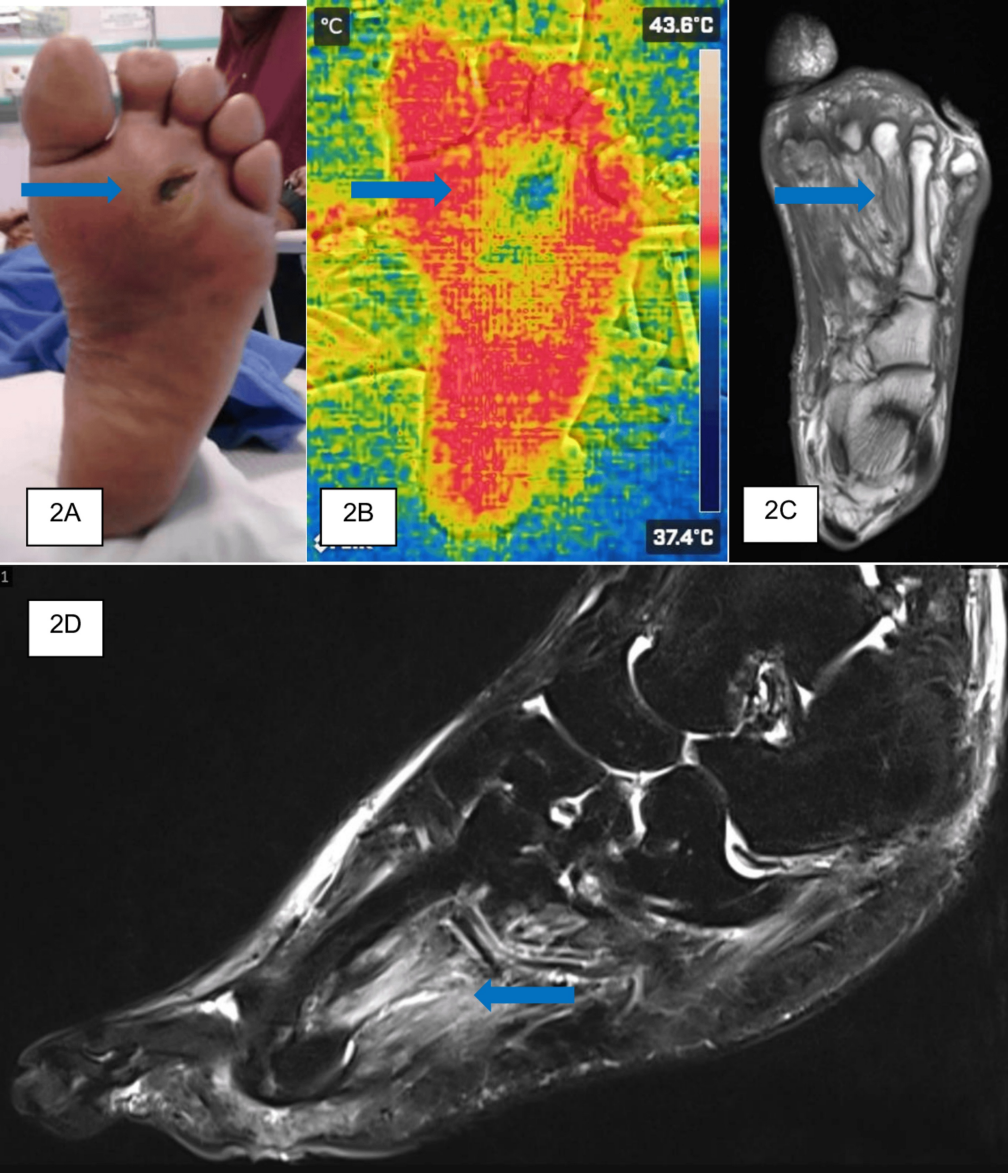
(A) Clinical figure of the affected foot, with the area of interest marked with the blue arrow. (B) Thermal imaging of the affected foot upon admission to the ward. (C) Axial view of the T1 MRI image of the affected foot. (D) Sagittal view of the T2 fat-saturation MRI image of the affected foot, with the border and depth of the infection clearly demarcated in the MRI image.
